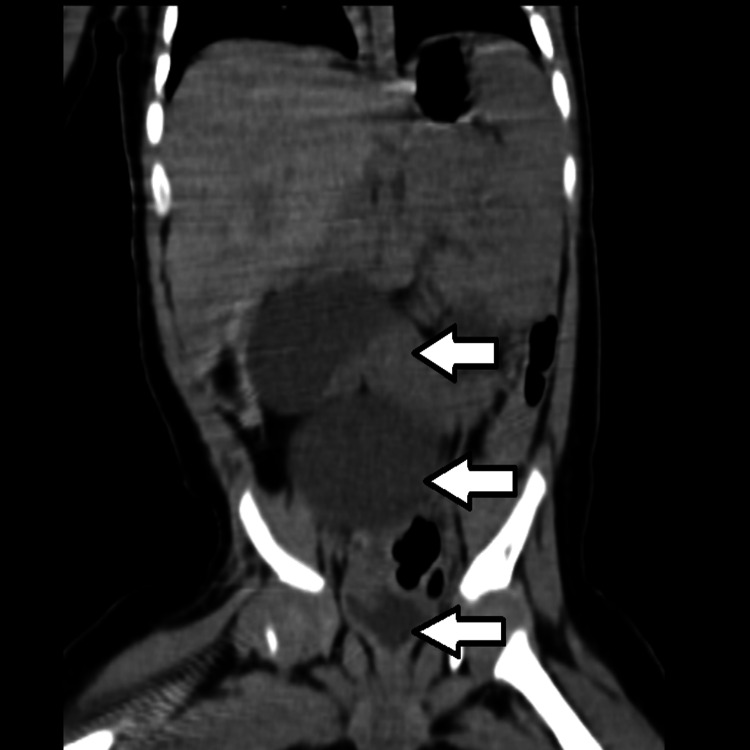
Coronal section of CT urography. Arrows show a grossly dilated renal pelvis with a severe right ureteric stricture and the bladder in a continuous sequence.
